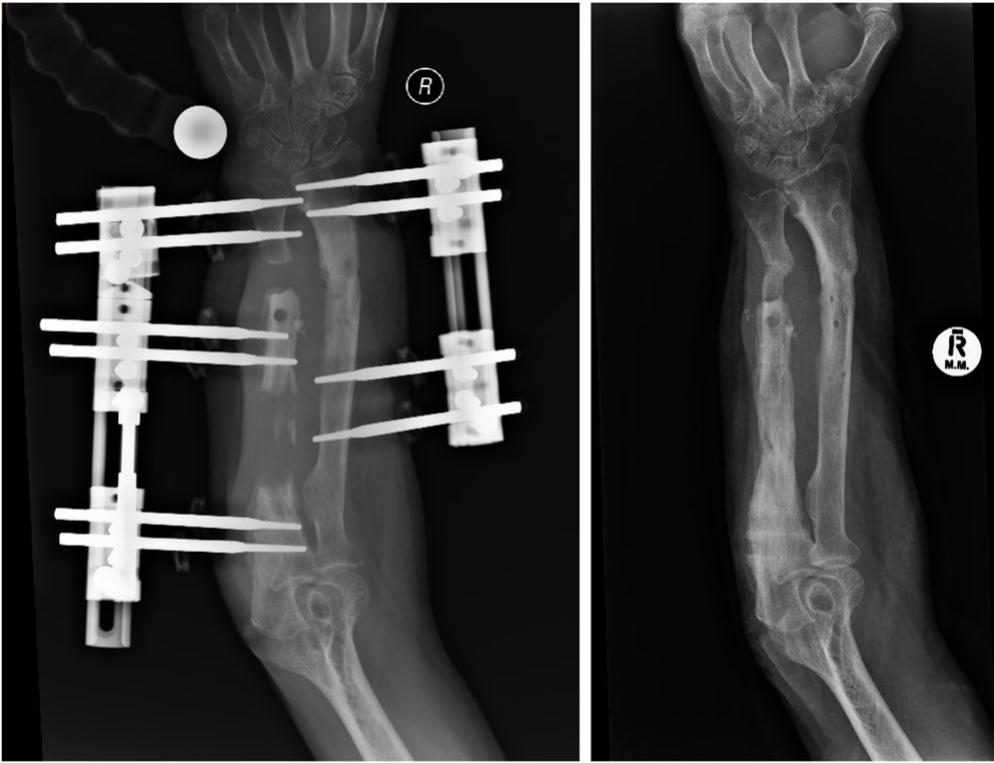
Distraction and outcome radiographs, Case 1
